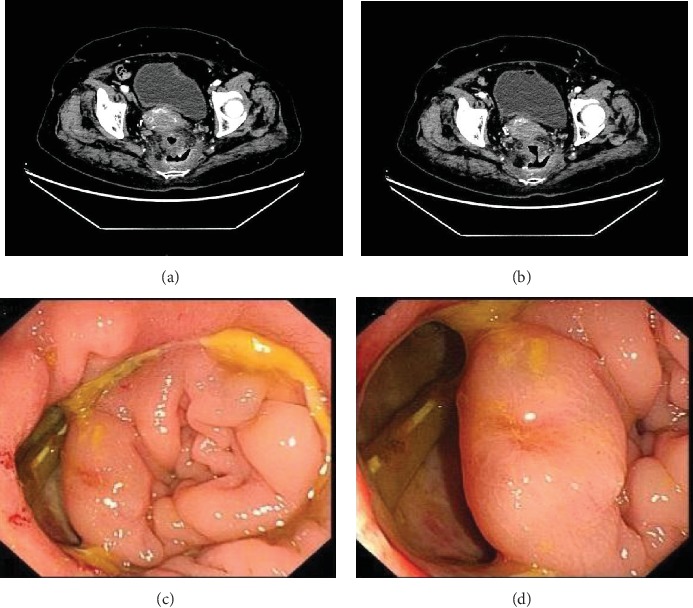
Images of anastomotic leakage. (a) Pelvic contrast-enhanced computed tomography picture showing pneumatosis and hydrops around the anastomosis; (b) pelvic contrast-enhanced computed tomography picture showing the communication between the lumen of the bowel and pelvic cavity; (c) endoscopic picture showing the defect of anastomosis; (d) endoscopic picture showing that the pelvic drainage tube can be seen from the anastomotic defect.
